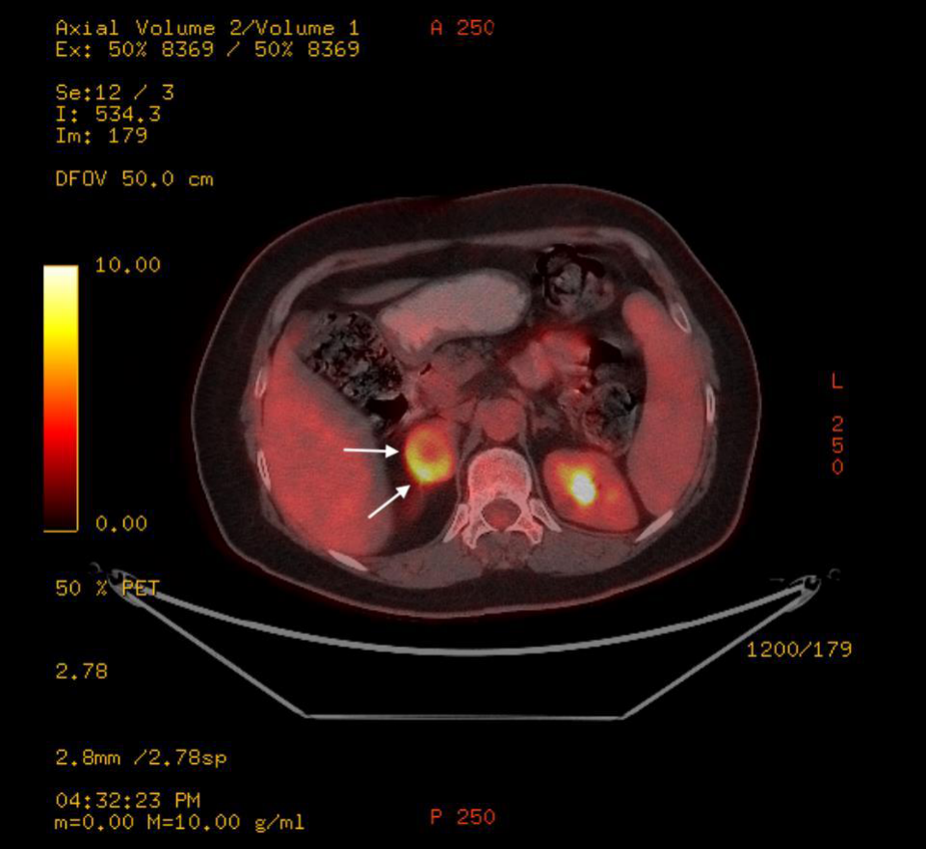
Right adrenal schwannoma in the FDG-PET/CT scan. This mass had an increased FDG uptake. The schwannoma was shown by a white arrow.
